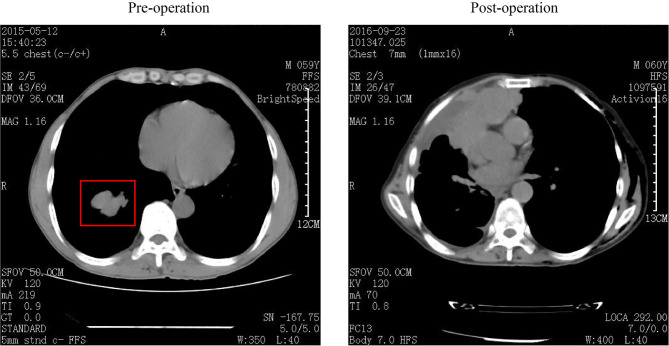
Computed tomography (CT) images of this patient. CT image was collected before (left) and after (right) surgery, and the tumor mass was labeled within the red box.
